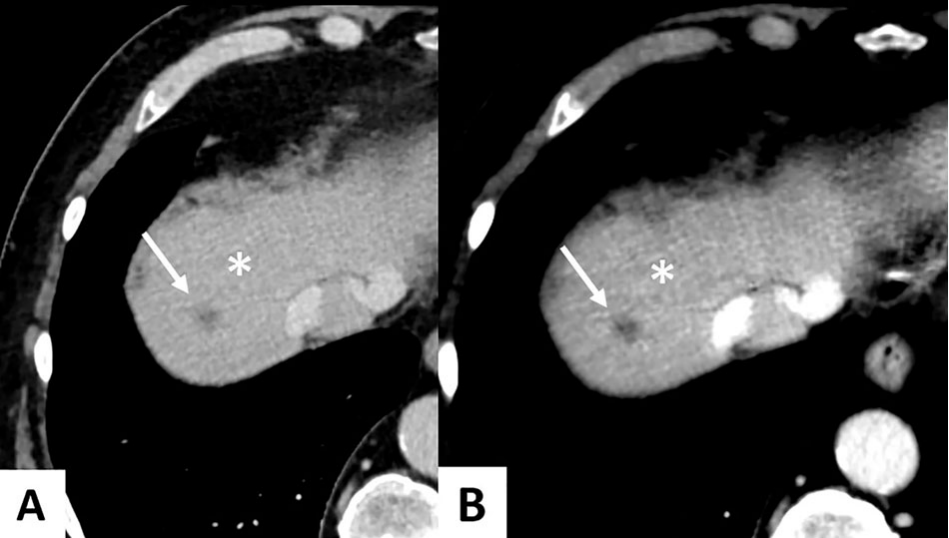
81-year-old patient with a recent diagnosis of right-sided colon adenocarcinoma and a single liver metastasis at baseline evaluation. DS-DECT (Siemens Force) in axial section at VP. A The conventional CT image shows a poorly defined 11 mm lesion (arrow) in the hepatic dome (star). B The 40 keV VMI image improves the contrast between the lesion (arrow) and the adjacent liver tissue (asterisk). Percutaneous biopsy of the lesion under CT guidance confirmed the metastatic nature of the lesion. CT, computed tomography; DS-DECT, dual-source dual-energy computed tomography; VMI, virtual monoenergetic images
